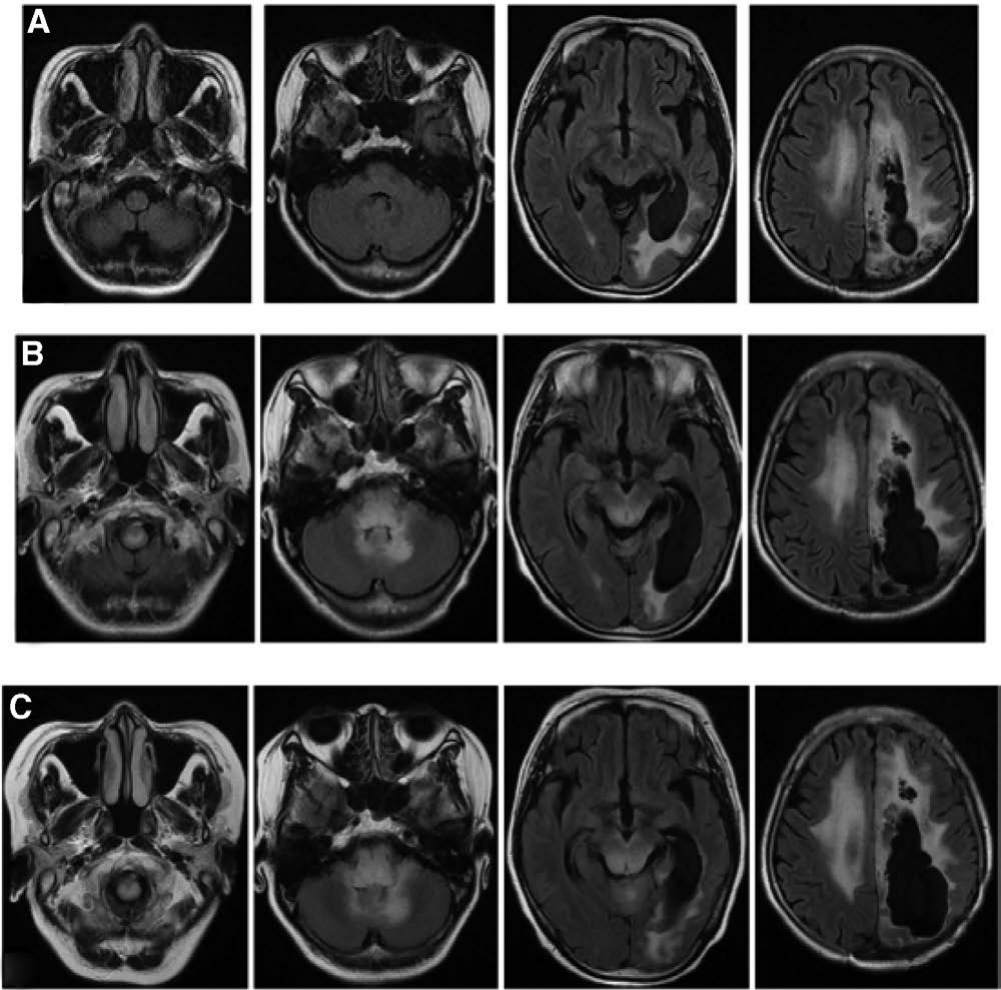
The T2 flair sequence of MRI. (1) Post-operation (A), this admission (B) and follow-up after discharge (C); (2) compared with post-operation, the brain stem and cerebellum developed diffuse hyperintensity at this admission; (3) compared with this admission, the brain stem and cerebellum developed more diffuse hyperintensity during follow-up.
